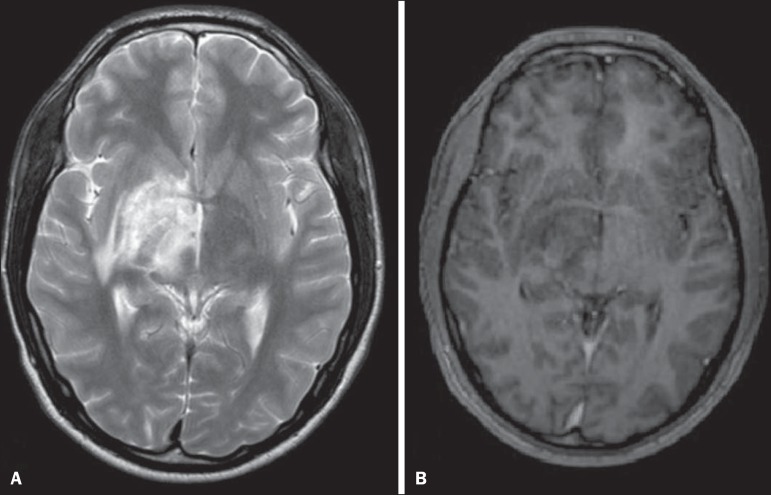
Neuro-Behçet's disease. MRI T2-weighted sequence
